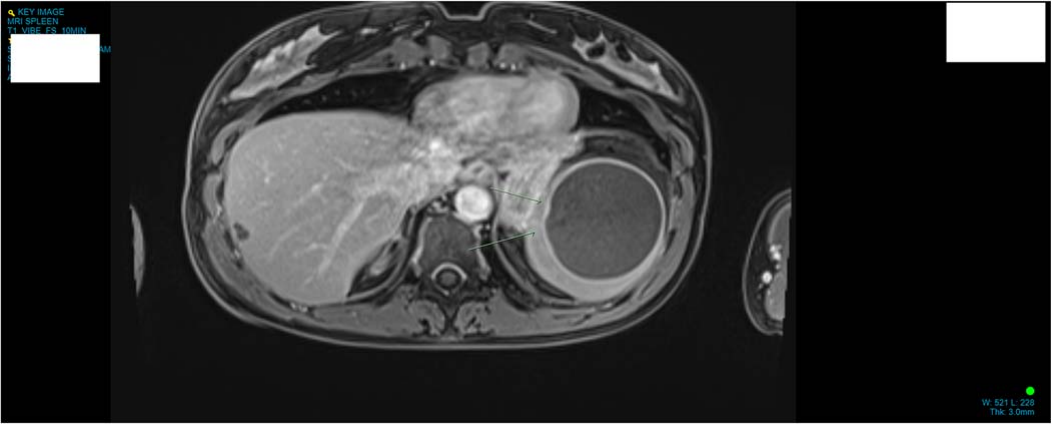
MRI demonstrating anatomical location and size of lesion sagittal plane.
